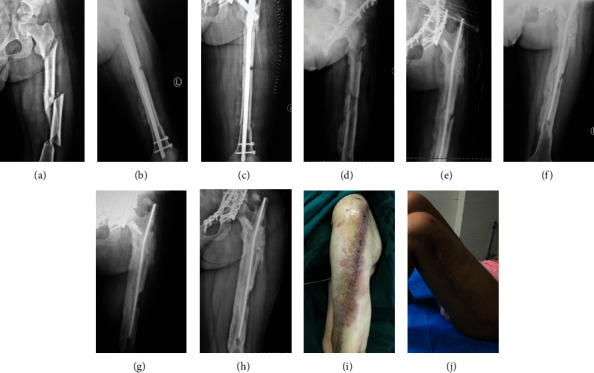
X-ray after the femoral shaft fracture (a), after internal fixation of femoral shaft fractures with the intramedullary nail (b), and at 6 weeks (c) postoperatively. After debridement and removal of internal fixation (d). After Kirschner wire open placement of bone cement (e), at 3 months (f), at 1 year (g), and at 2 years (h). General images before (i) and after (j) treatment.
